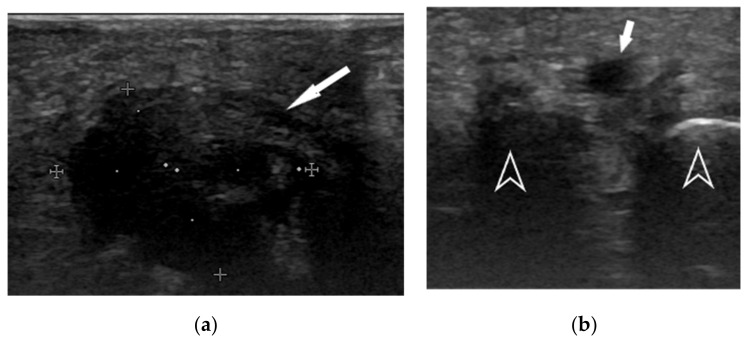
Dynamic ultrasound (a) showing a neuroma of the III intermetatarsal space (arrow). During Mulder’s test (b), the lesion (arrow) is pushed superficially, thus becoming apparent between the adjacent metatarsal heads (arrow heads).
